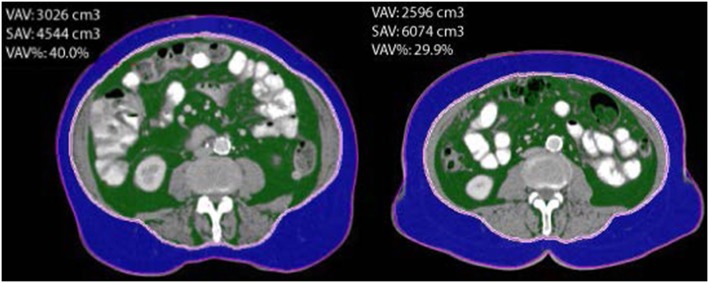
Representative examples of CT scan results. Visceral fat in green and subcutaneous fat in purple. CT scan on the left with high VAV, CT scan on the right with high SAV
